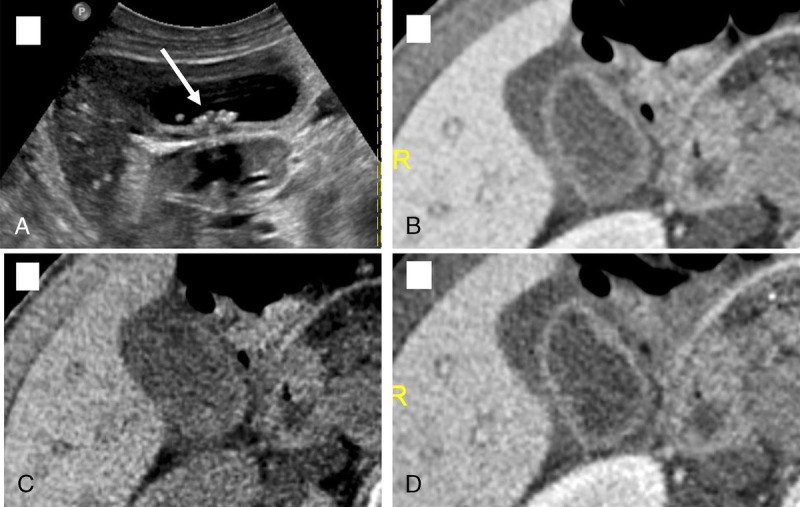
False negative: No GSs was seen by either of 4 reviewers on PC (B), VNC (C) or 50-keV VMC (C) images. Ultrasound performed (A) 2 weeks ago showed definite GSs.
